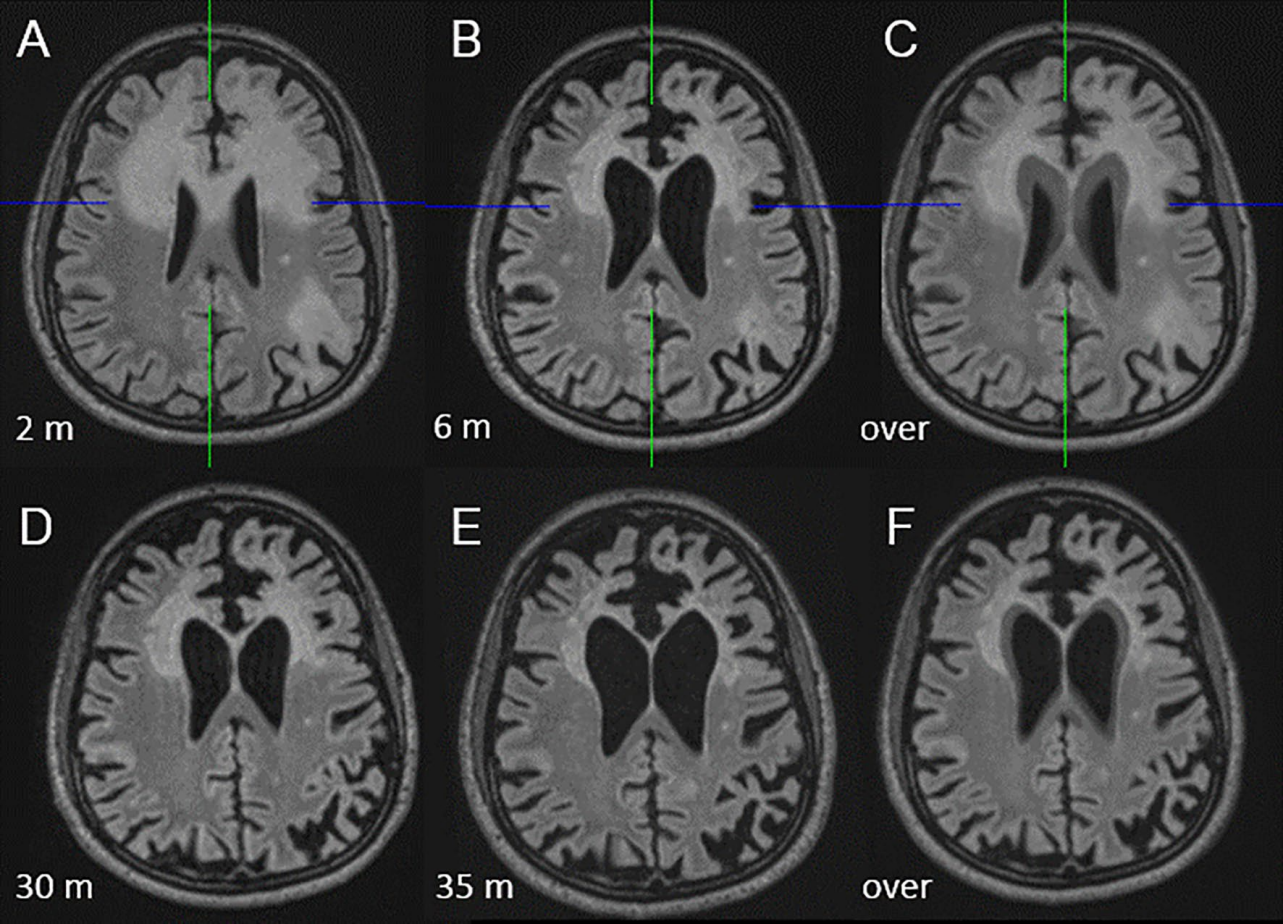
Shrinking PML lesion during therapy and progressive atrophy in Patient 5. FLAIR images showing the largest lesion extension (A) after two months. B Shrinkage of the lesion four months later (B), previously published in [21]. (D) and (E) showing progressive tissue atrophy between 30 and 35 months after commencement of pembrolizumab. C is an overlay of A and B, (F) is an overlay of (D) and (E) with 50% transparency each. *m* indicating months after the first administration of pembrolizumab, *over* overlap
